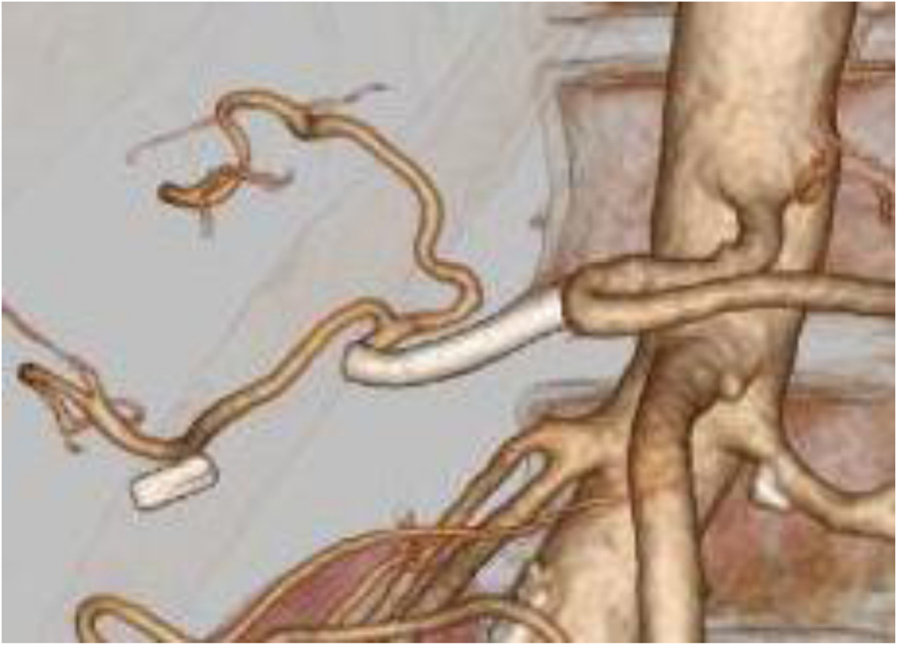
CT arteriography performed 10 months after stent graft placement, which indicated the patency of the stent graft without stenosis of distal hepatic arteries
